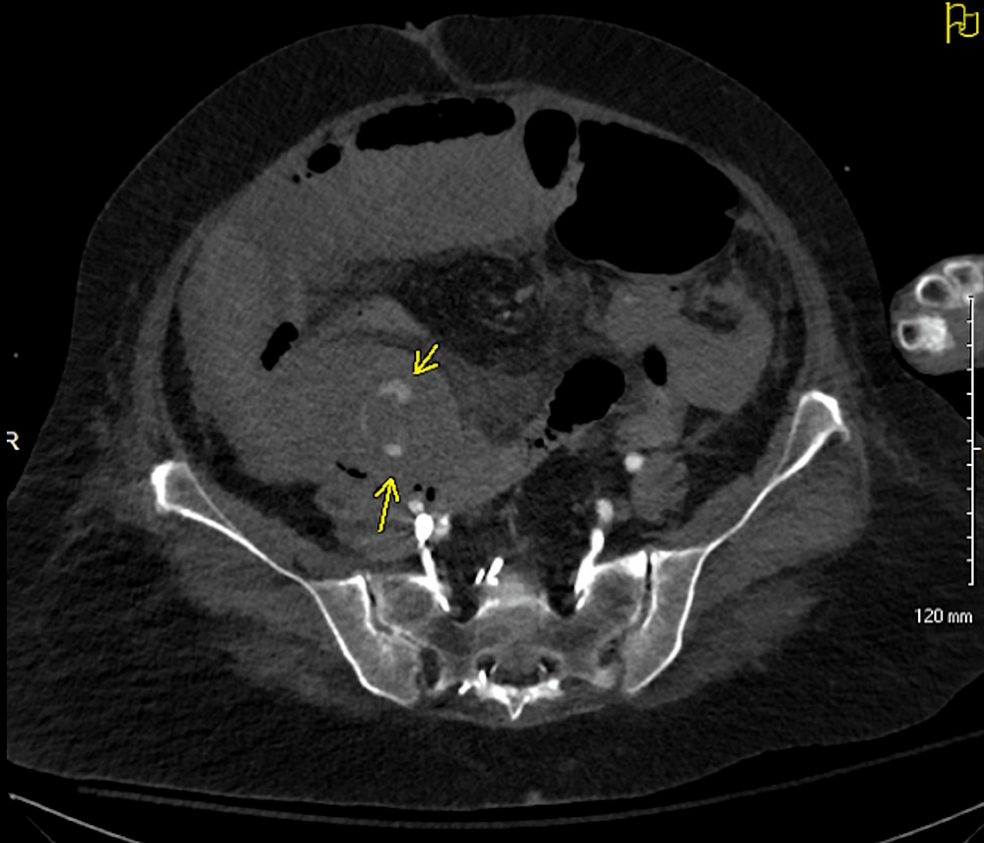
Contrast-enhanced (IV only) CT of the abdomen and pelvis (axial view) demonstrating evidence of intramural hematoma (arrow)
